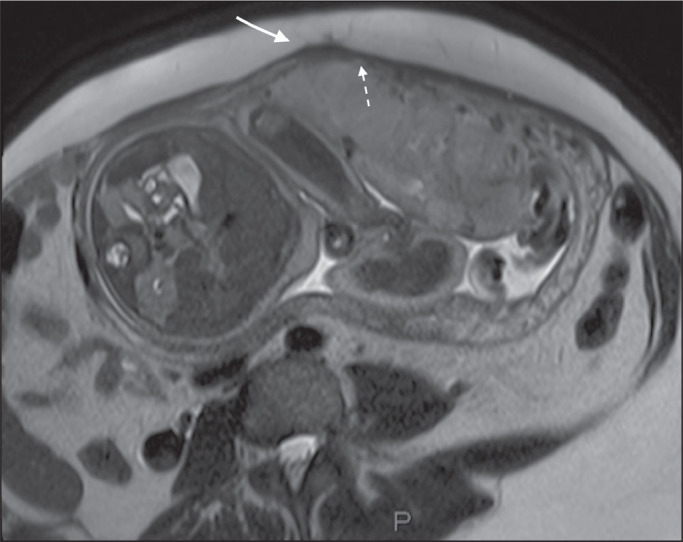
A 36-year-old patient with placenta percreta. Axial T2-weighted HASTE
